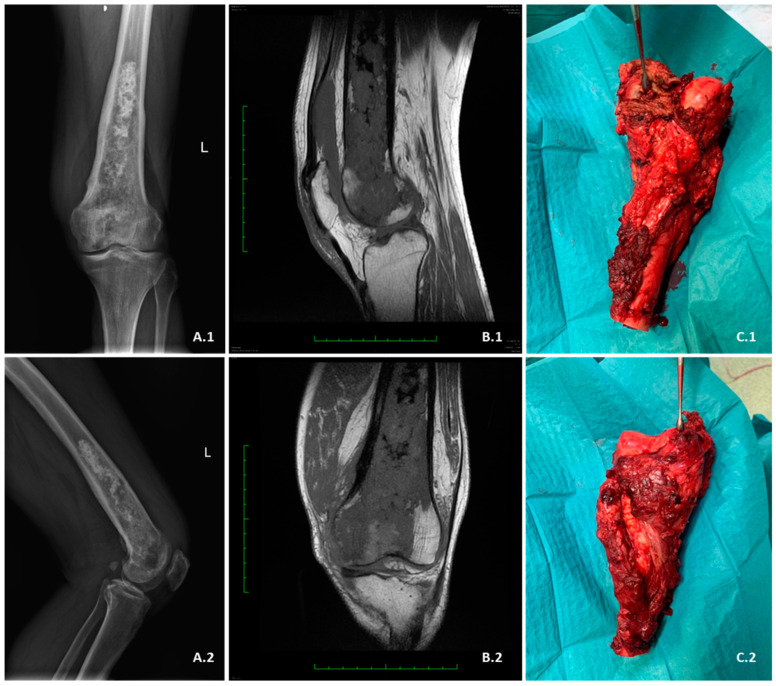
WHO G3 dedifferentiated chondrosarcoma of distal femur. (A.1,A.2)–AP/lateral (LAT) X-ray preoperative views. (B.1) Saggital fat saturated T1 MRI view. (B.2) Coronal fat saturated T1 MRI view. (C.1,C.2) Intraoperative views of the resected distal femur.
